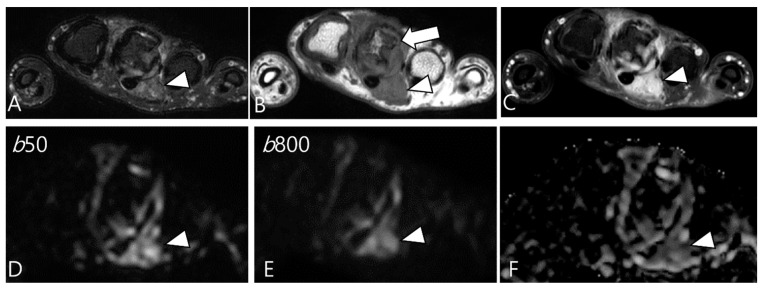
A 37-year-old female with D-TSGCT in the hand. (A) Axial fat-suppressed T2WI shows lobulated soft tissue with heterogenous SI around the third MCP joint (arrowhead). (B) Axial T1WI shows the mass with iso SI (arrowhead) and marked extrinsic bony erosion at the metacarpal bone (arrow) is noted. (C) Axial contrast-enhanced fat-suppressed T1WI shows the homogeneous enhancement of the mass (arrowhead). (D,E) Due to microcapillary perfusion, the mass shows an apparent high SI on the low b-value image of the DWI (arrowhead) compared to the high b-value image of the DWI. (F) The mass shows a low ADC value (arrowhead), suggesting diffusion restriction.
